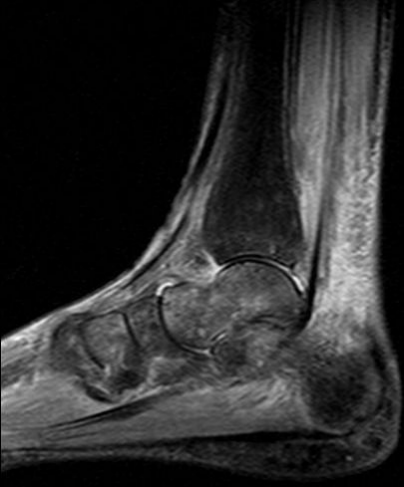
Same foot as in Figure 1. MRI sagittal STIR-sequence shows edema of skin and subcutaneous tissue with diffuse and patchy bone marrow edema in the calcaneus, talus, distal tibia and in the anterior tarsus (CRPS I).
